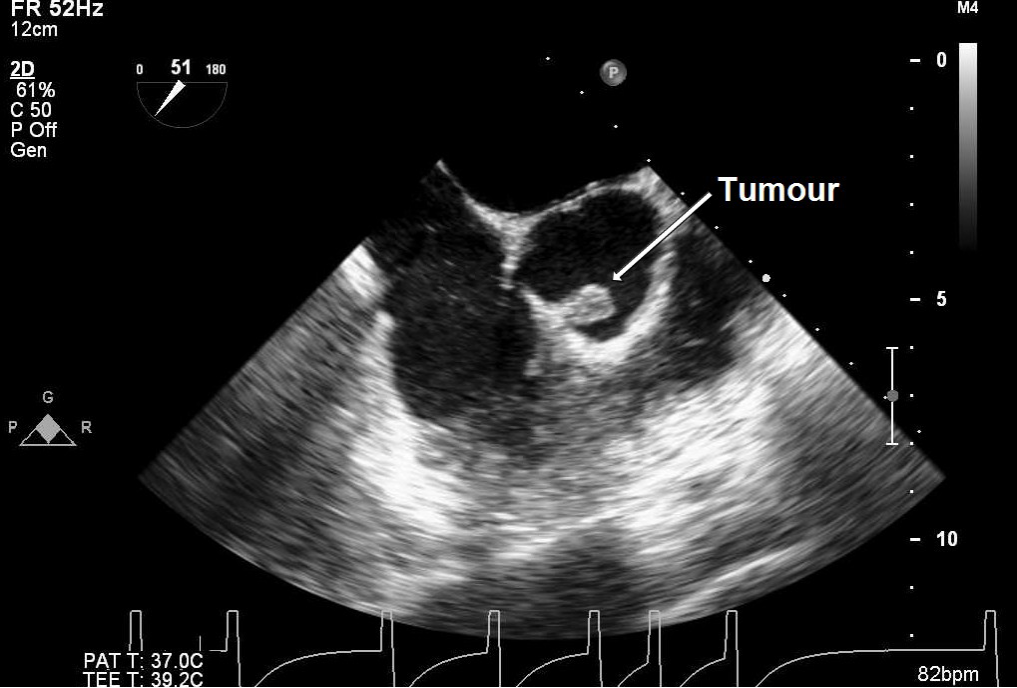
Trans-oesophageal echocardiography showing a mobile, spherical pedunculated tumour mass of 1.2 × 1 cm in size at the right coronary aortic cusp (ME AV short-axis view).
